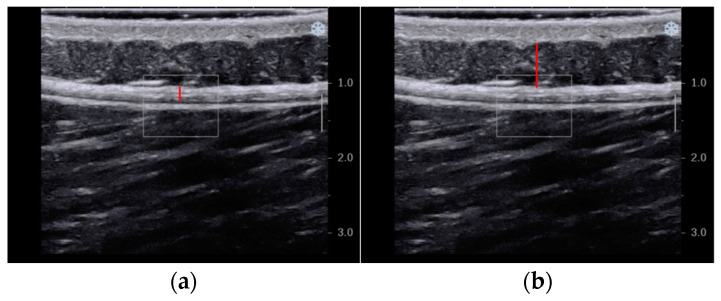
Thickness of the tissues in ultrasound images: (a) TLF and (b) subcutaneous tissue.
